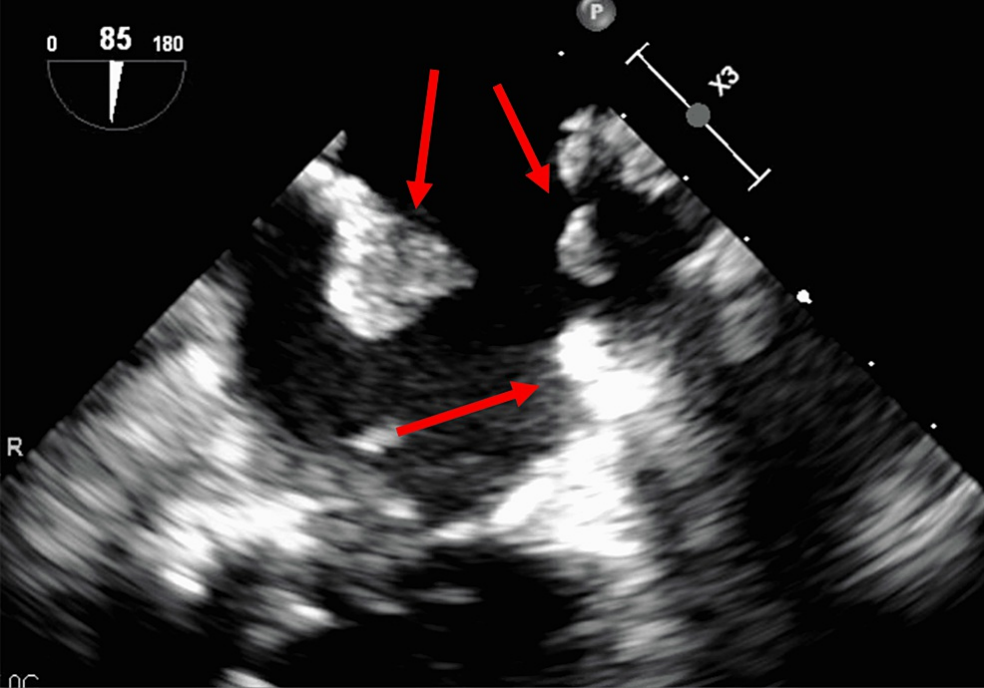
Transesophageal echocardiography (TEE) showing multiple atrial myxomas (red arrows)
